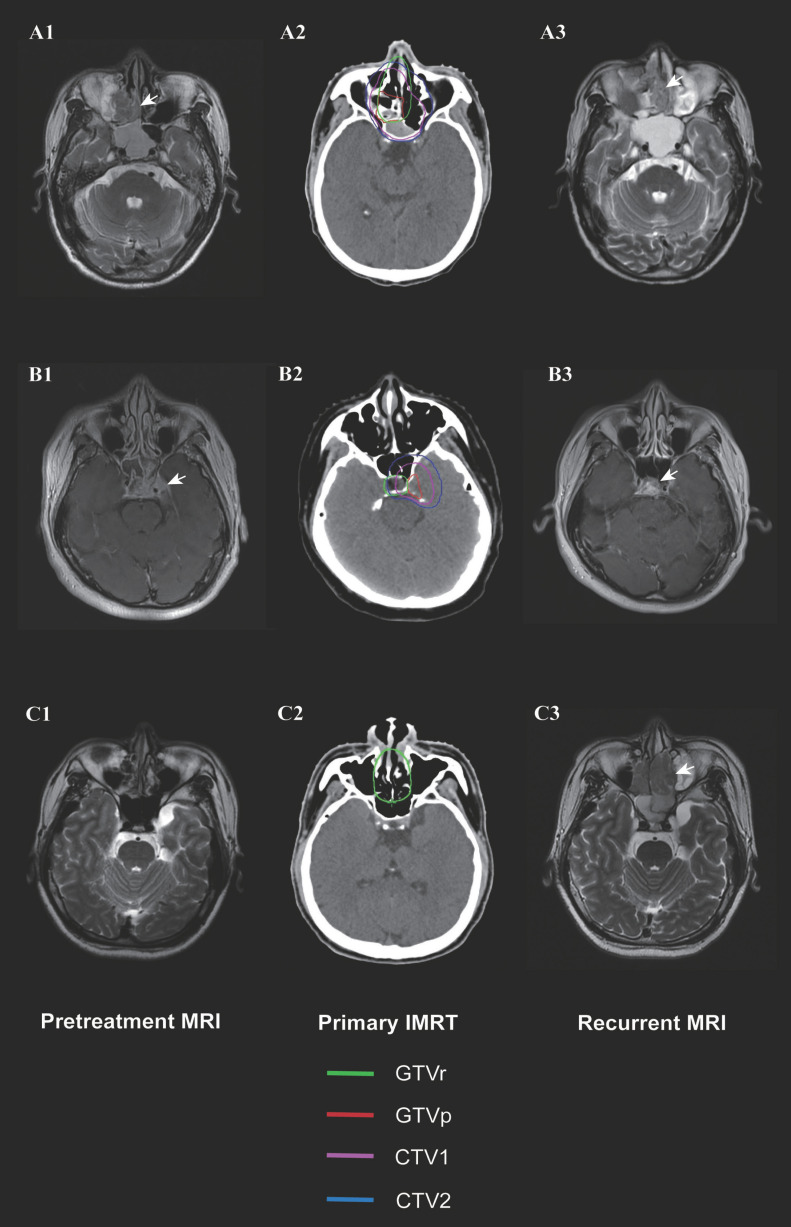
Magnetic resonance imaging (MRI) images of three patients with local recurrence outside the nasopharynx. Marginal failure in the ethmoid sinus and nasal cavity due to the large volume of the recurrent tumor (A1-A3); marginal failure at the hypophyseal fossa due to target miss (B1-B3); and out-field failure in the ethmoid sinus and nasal cavity (C1-C3). Left: Initial diagnostic MRI. Middle: Target volume delineation and dose prescription of primary intensity-modulated radiotherapy. Right: MRI at recurrence. The green line indicates the gross recurrent tumor volume (GTVr); the red line, the gross primary tumor volume (GTVp); the pink line, the high-risk clinical target volume (CTV1); and the blue line, the low-risk clinical target volume (CTV2).
